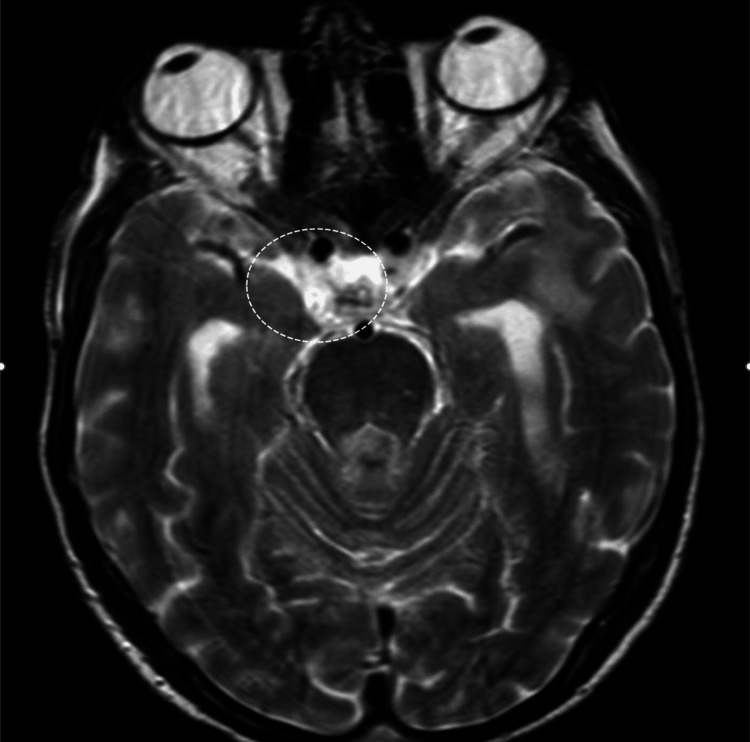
Brain MRI showing leptomeningitis with diffuse leptomeningeal enhancement and pachymeningeal enhancement extending into the right cavernous sinus.
